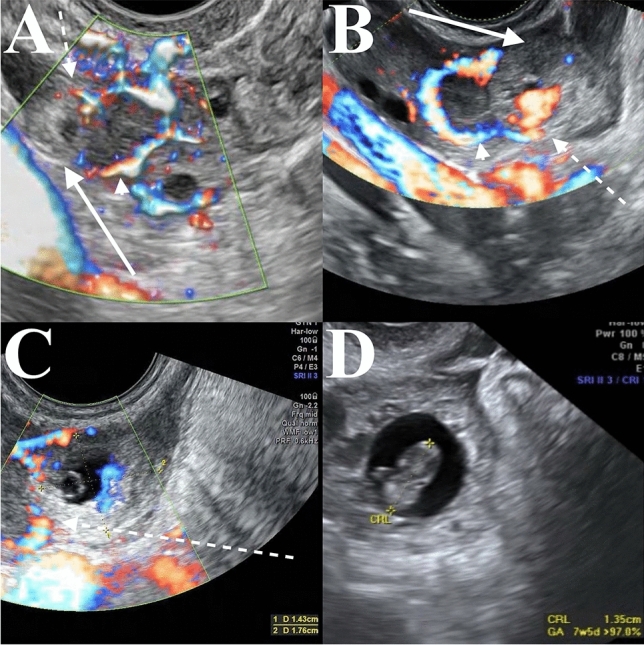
Sonographic images of Cases 3, 5, 7, and 13. *Arrow indicates the ovarian pregnancy. *Arrowheads indicates the corpus luteum. *Dotted arrow indicates the dominant-vessel Doppler sign leading to the ovarian pregnancy. *A Case 3, GA of 4 + 6W, β-hCG 2161, OP with CL; B Case 5, GA of 5W, β-hCG 4941. OP with CL; C Case 7, GA of 6 + 1W, β-hCG 12787. OP with yolk sac; D Case 13, GA of 9 + 4W (CRL = 7 + 5W), β-hCG 19647
